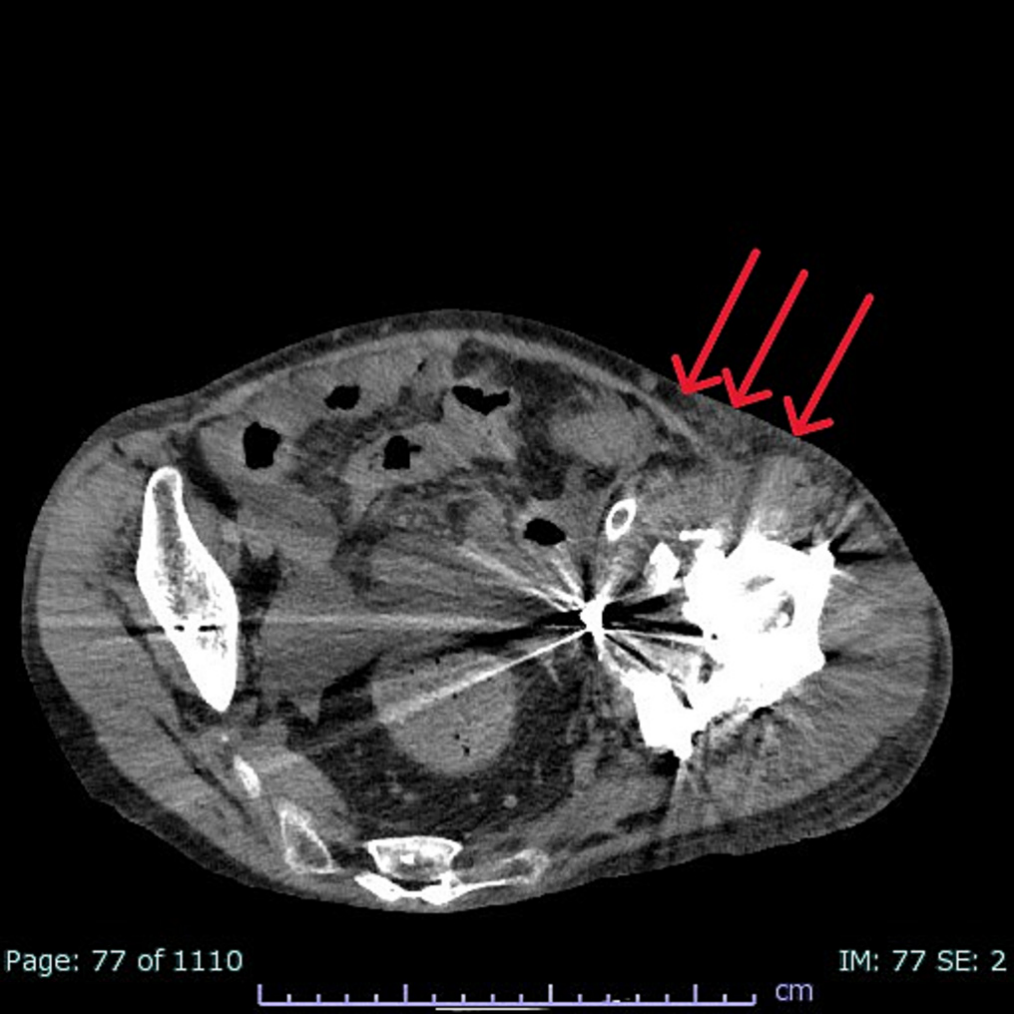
Follow-up computed tomography showed no re-expansion of the external iliac artery aneurysm or rupture, and the implanted stent graft was patent and well positioned.
